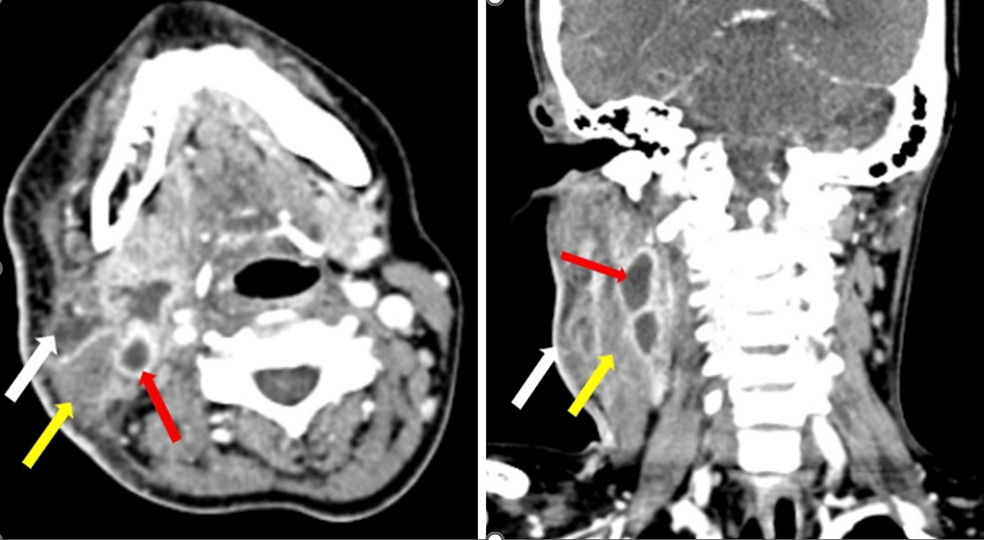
(Case 3) Axial and coronal contrast-enhanced CT of the neck reveals a bizarre-shaped hypodense fluid collection involving the superficial and deep facial planes of the neck on right side. The fluid collection shows a predominantly absent peripheral rim of enhancement (white arrows). However, a thick rind of enhancement is seen surrounding few fluid pockets suggesting abscess formation (red arrows). The right sternocleidomastoid muscle belly appears bulky and shows reduced enhancement as compared to the opposite side, suggesting myositis (yellow arrows).
